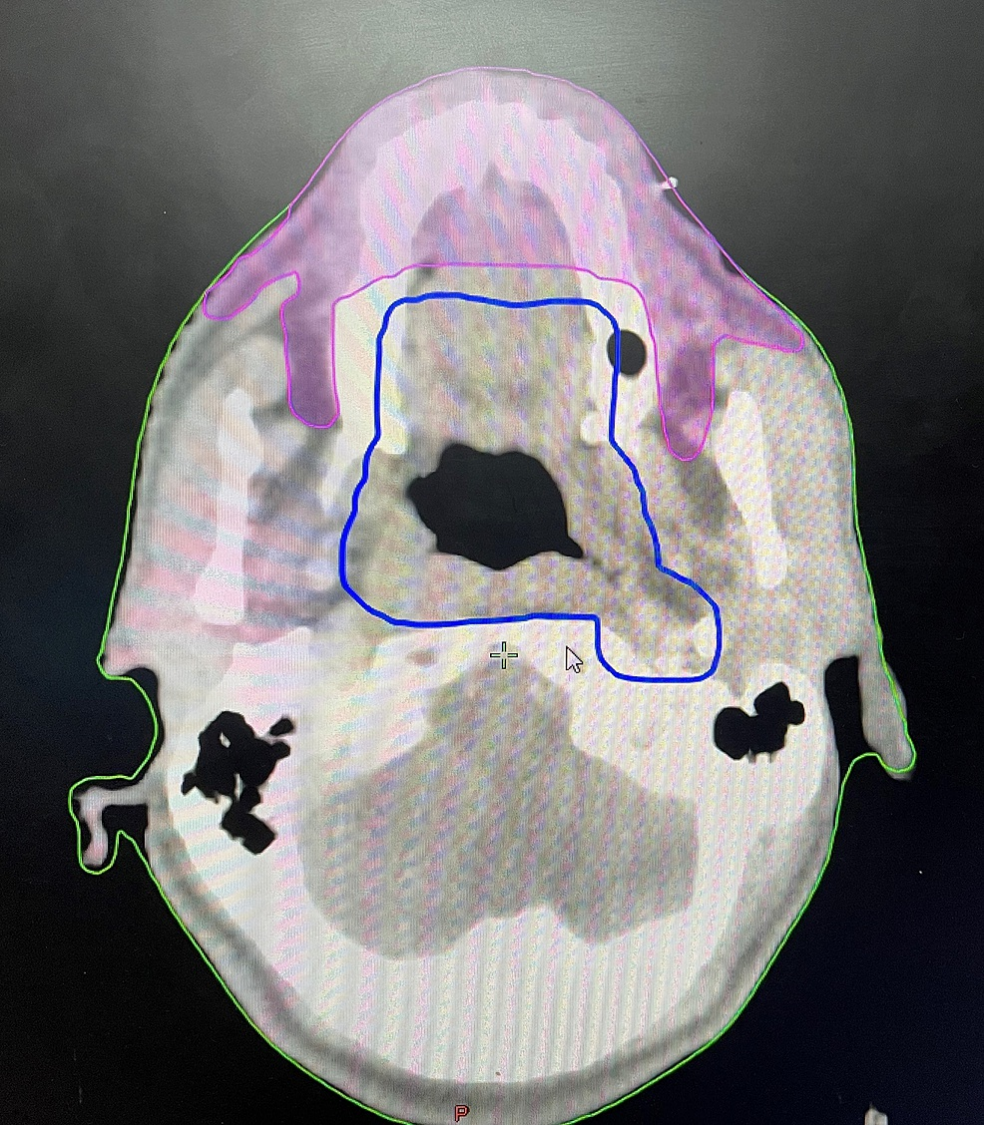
Cross-sectional CT scan image showing the delineation of PTV (blue) and pseudo-OAR (pink) CT, computed tomography; PTV, planning target volume; OAR, organ at risk
